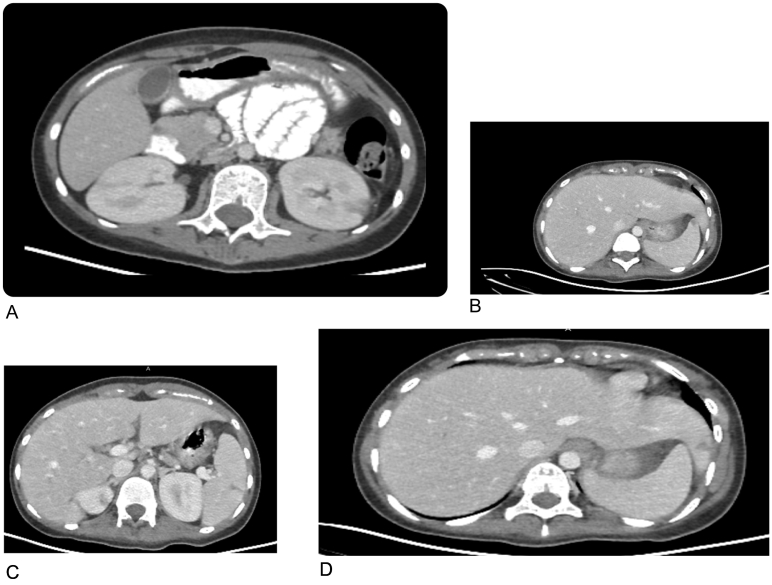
A: Axial CT scan with IV and oral contrast portal-venous phase: shows; wedge shaped hypodense areas in both kidneys, the largest on the left side measuring about 1.3 × 0.8 cm representing bilateral renal infarcted areas. B: Axial CT scan with IV contrast in portal venous phase: Two small hypodense areas are seen within the spleen measuring 0.4 ∗ 1 cm. C: Axial CT scan with IV contrast in portal venous phase: Two small hypodense areas are seen within the spleen measuring 0.7 ∗ 1 cm. D: Axial CT scan with IV and oral contrast portal venous phase: small hypodense area triangular shape seen in the left liver lobe measuring about 1 ∗ 0.6 cm presenting a focal small infarcted area.
